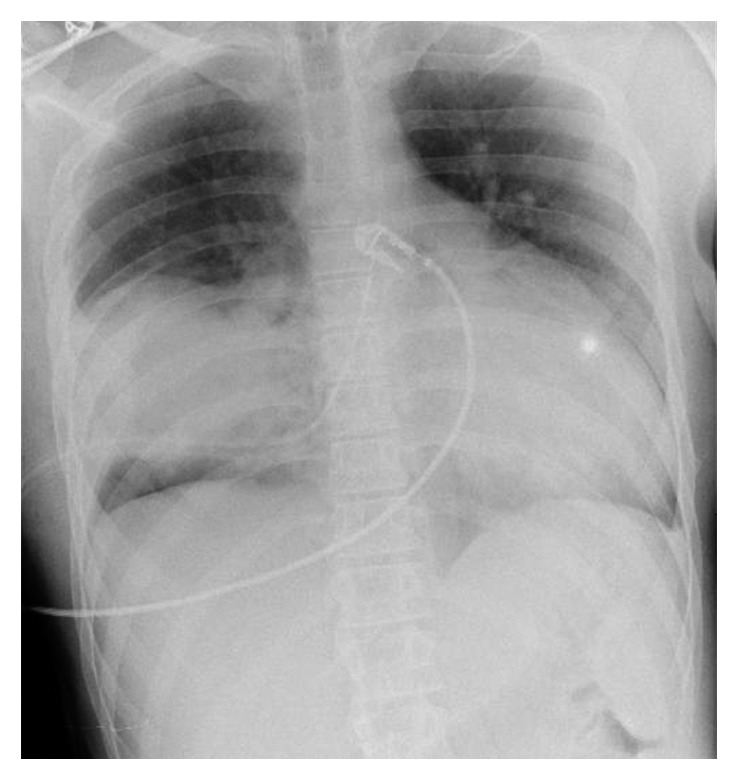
Thoracic radiograph with thoracic drain.
